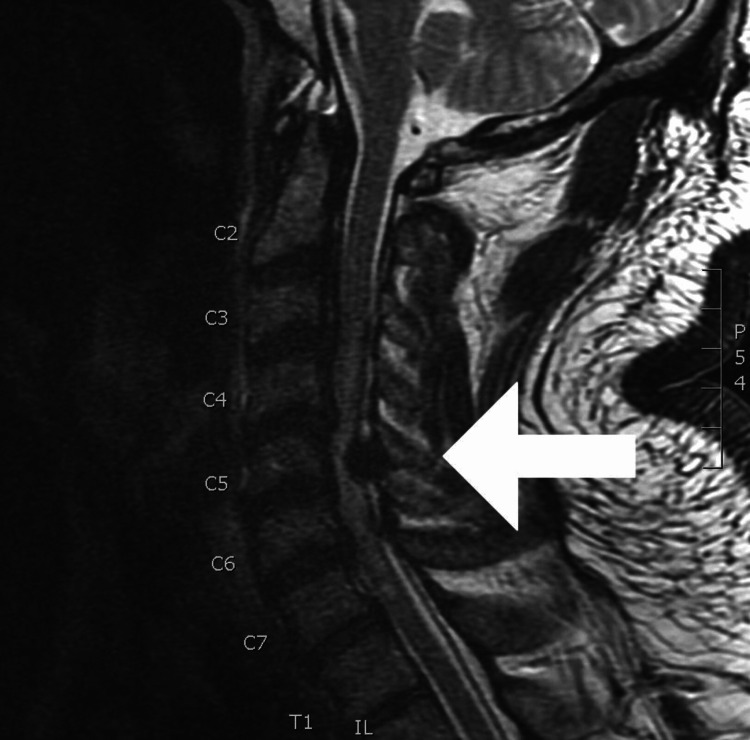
T2-weighted cervical spine MRI exhibiting evidence of chronic spinal canal stenosis with complete cord flattening at the C5 level with profound cord edema centered at the C5 level extending from C3 to T1. MRI: Magnetic resonance imaging
